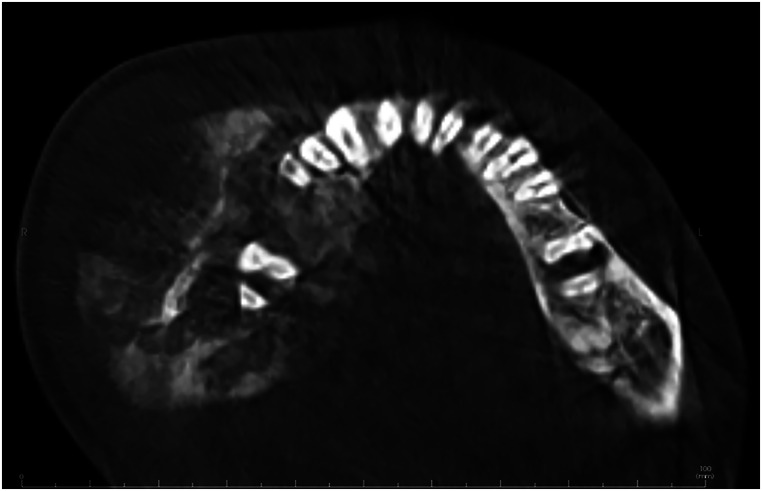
Cone beam computed tomography image shows an intramedullary ill-defined and destructive mass in the right body of the mandible, with extensive periosteal reaction (sunburst appearance) and soft tissue component
